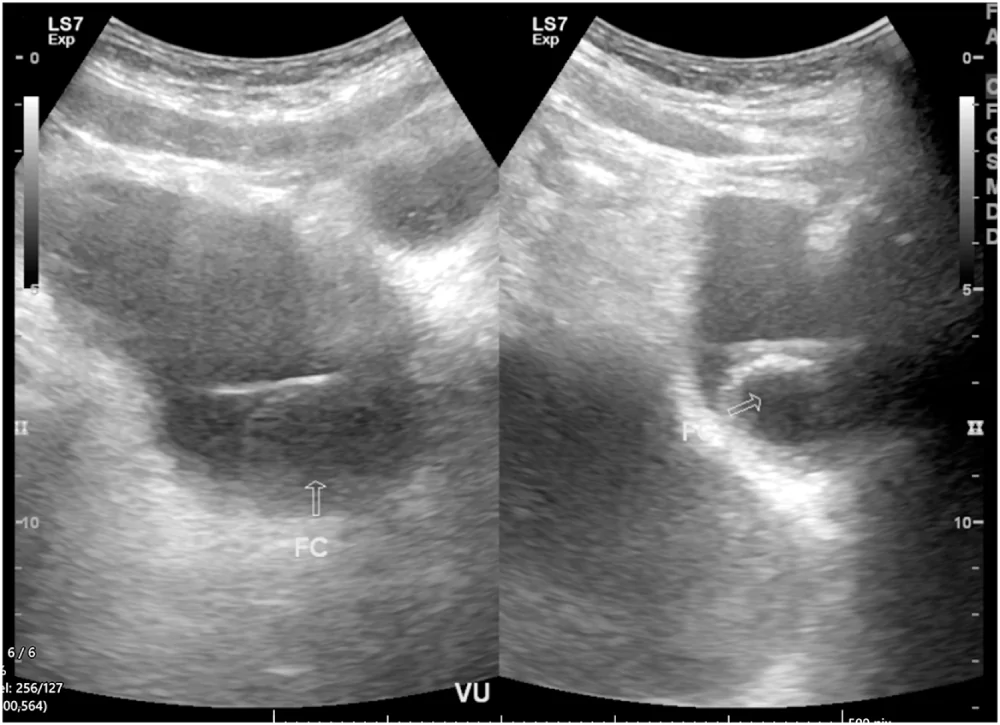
Abdominal ultrasound showed a well-defined hypoechoic fluid collection (FC) with internal debris consistent with a peritoneal abscess formation at the left suprapubic paramedian region.
